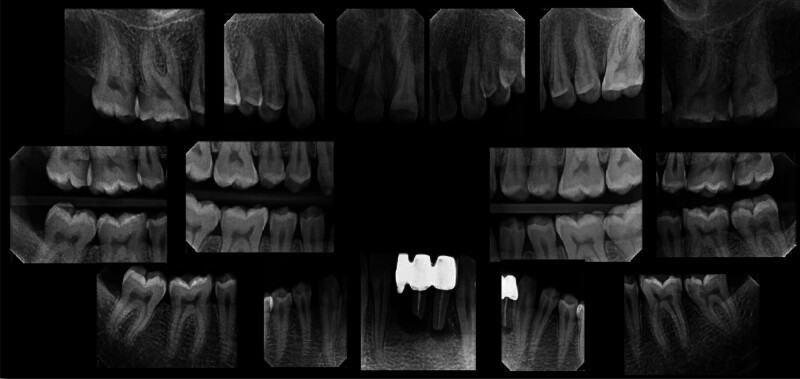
Preoperative full-mouth X-rays.
